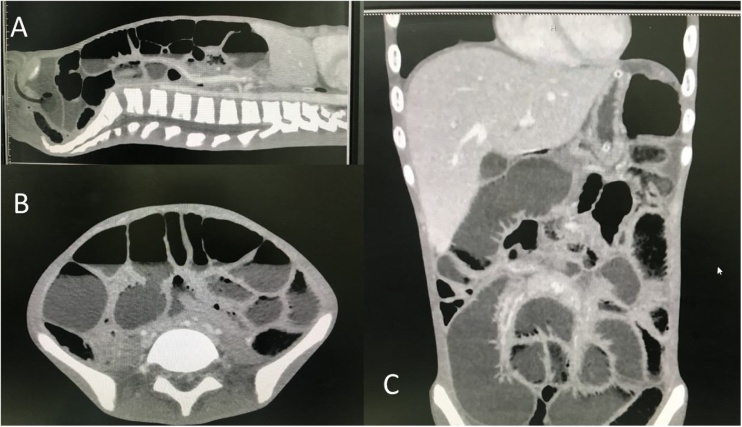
Sagittal (A), axial (B), and coronal (C) CT scan views showed the same findings as shown in radiography.
